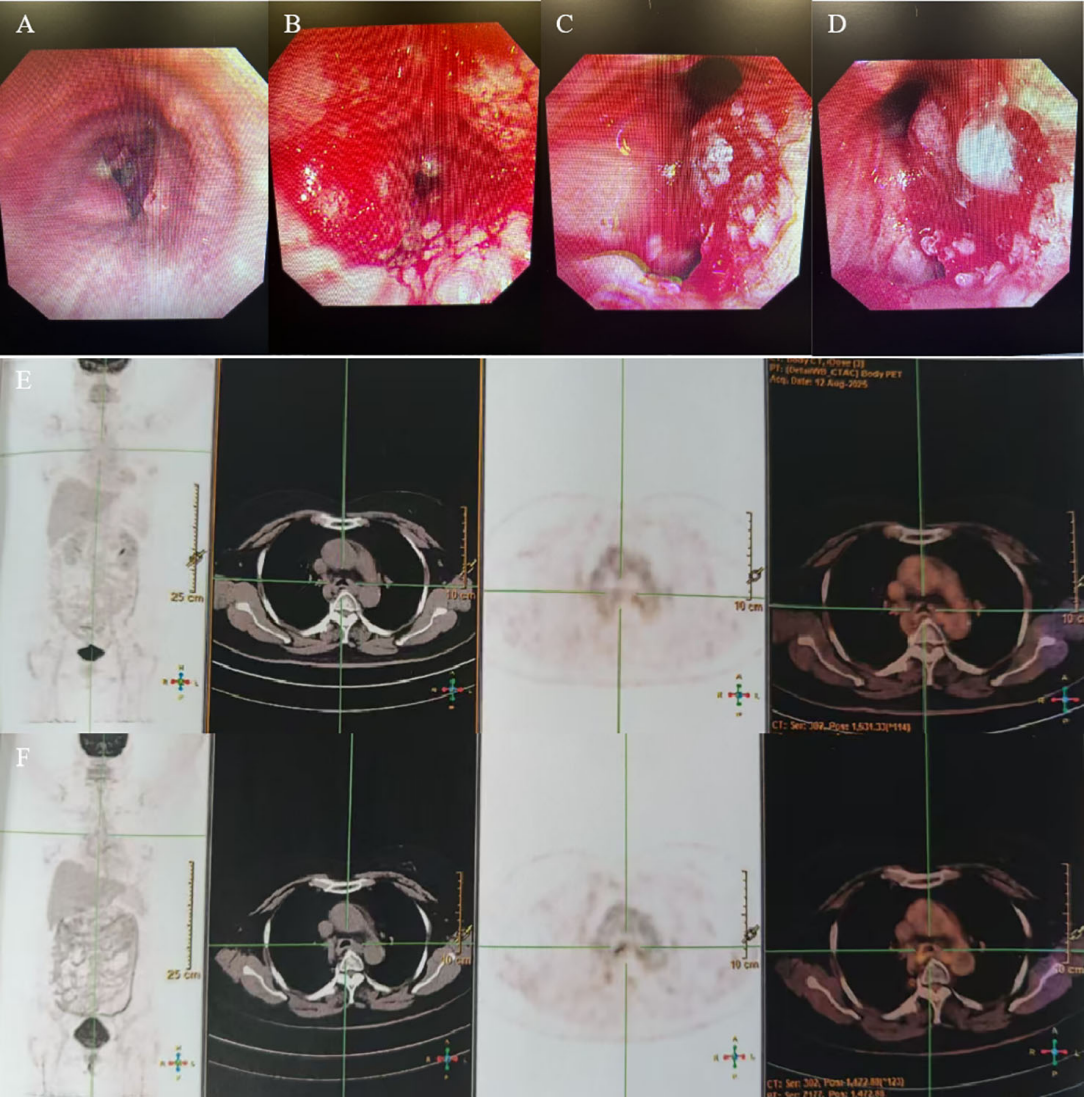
(A–D) Fiberoptic bronchoscopy showed a trachea lesion situated 3 cm above the carina, and the lumen appeared notably narrow. (E, F) PET/CT revealed the thickness of the tracheal carina, showing no 18-FDG uptake and without distant metastasis.
